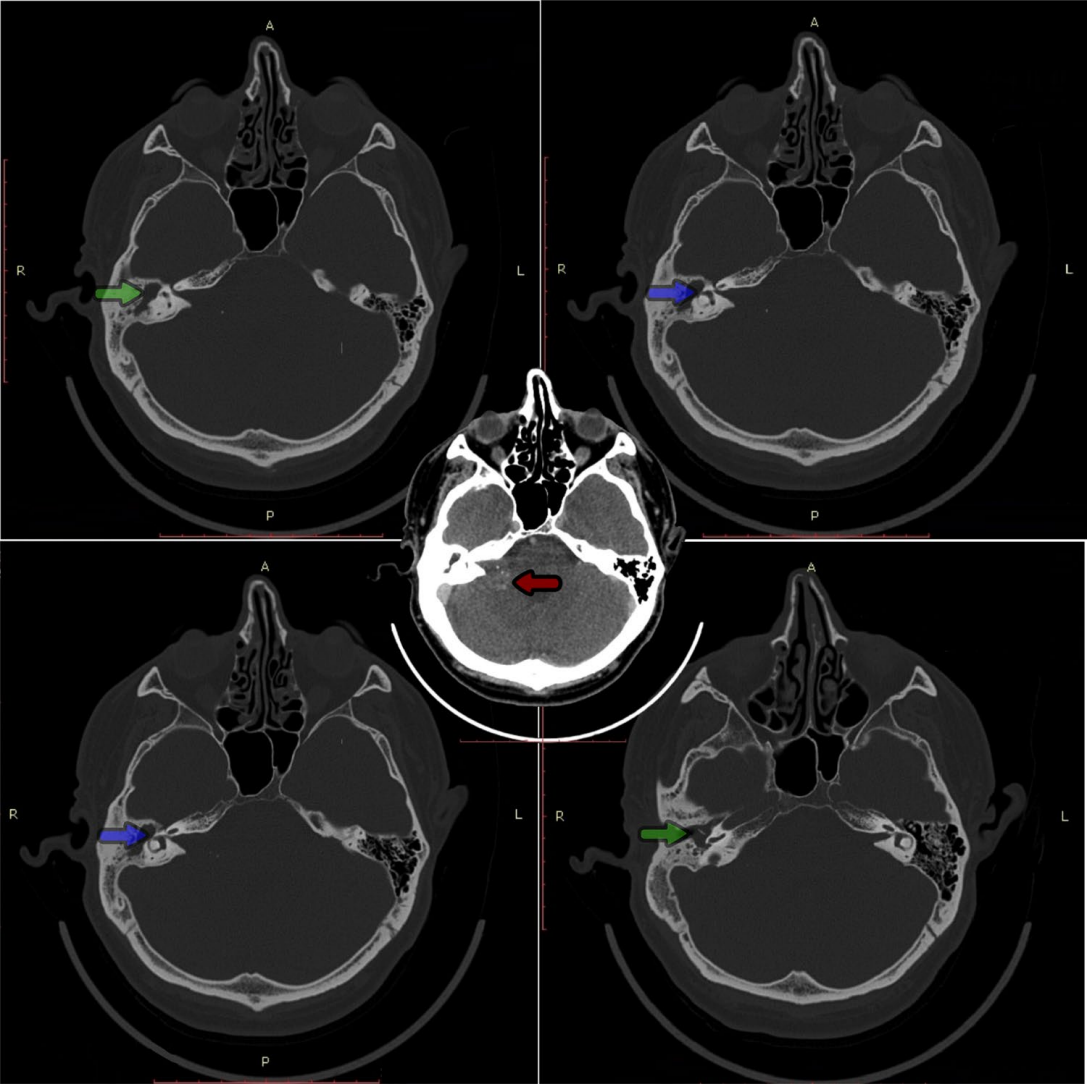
HRCT scan of the ears (before surgery). On the centre scan pathology of the middle fossa—right CP-angle tumor—red arrow. On the surrounding scans—pathology of the middle ear. Cholesteatoma of the tympanic cavity and mastoid (green arrow), lateral semicircular canal fistula (blue arrow)
